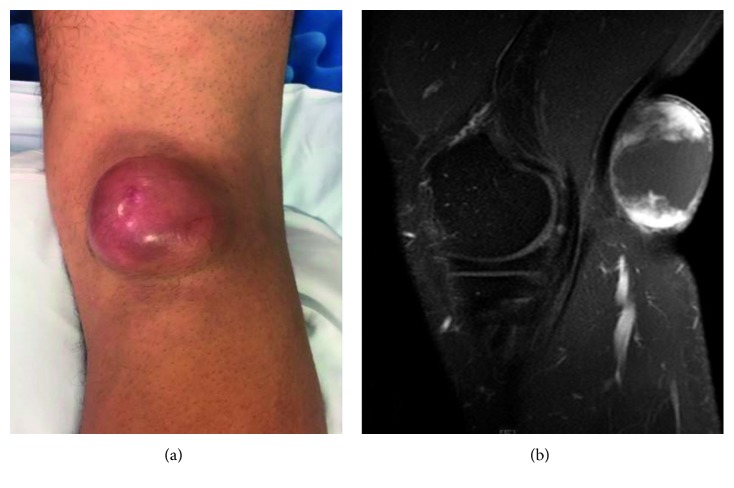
The image shows a 40-year-old male with a painless, superficial popliteal mass seen on (a) physical examination and (b) magnetic resonance imaging. In-office biopsy results showed intermediate-grade soft tissue sarcoma of indeterminate type. Final surgical pathology results showed grade 2 fibrosarcoma.
